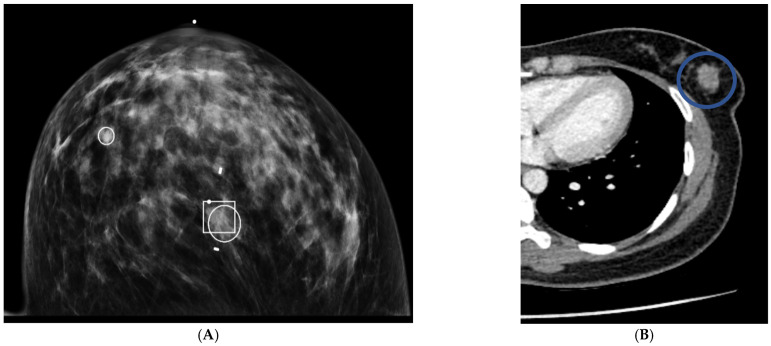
Craniocaudal mammographic image reveals clustered left breast microcalcifications that were recommended for biopsy (A). Contrast chest CT in axial projection on the same day reveals a 20 mm mass with an average HU of 61 (B). Biopsy results were invasive breast cancer.
